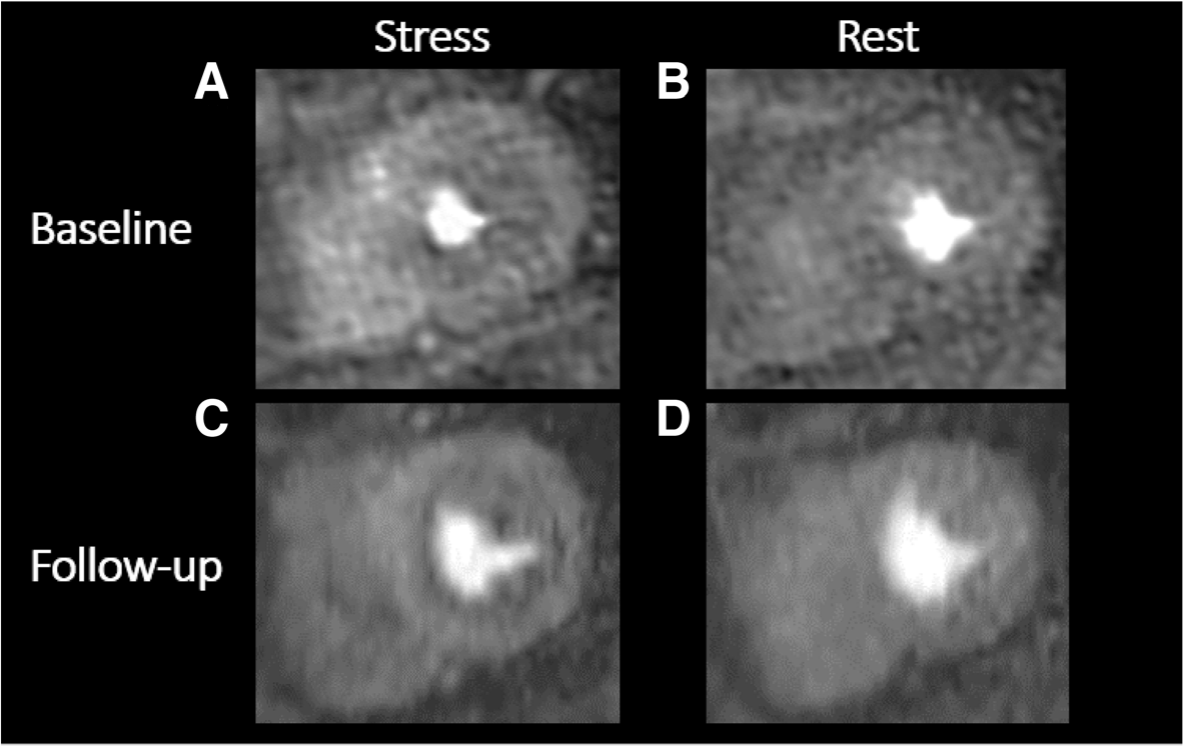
Baseline (a, b) and 10-year follow-up (c, d) adenosine stress first-pass perfusion cardiac magnetic resonance imaging showing evidence of circumferential subendocardial hypoperfusion at stress, consistent with coronary microvascular dysfunction-related ischemia. Myocardial perfusion reserve index decreased from 1.8 to 1.1 over 10-year period, indicating worsened ischemia
